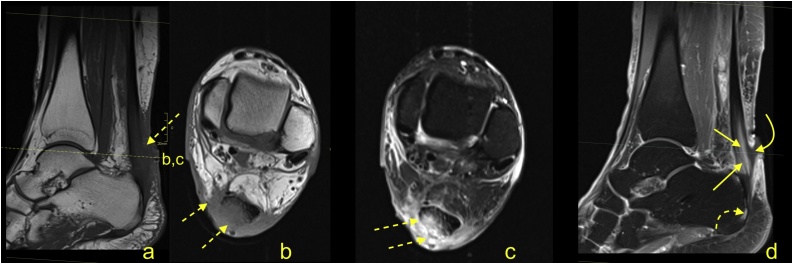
Infection of the Achilles tendon because of a non-healing wound on the heel in a 68-year-old patient. MRI (a-d) revealed extensive edema of the skin and subcutaneous tissue (dashed arrow) around the fistula (curved arrow). After contrast administration, enhancement is visible in the Achilles tendon (straight arrow) at the fistula level. A small area of bone marrow edema in the calcaneus (curved dashed arrow) is probably not related to inflammation. A- T1- weighted, b- T1- weighted with contrast, c and d- T1- weighted with fat suppression and contrast.
